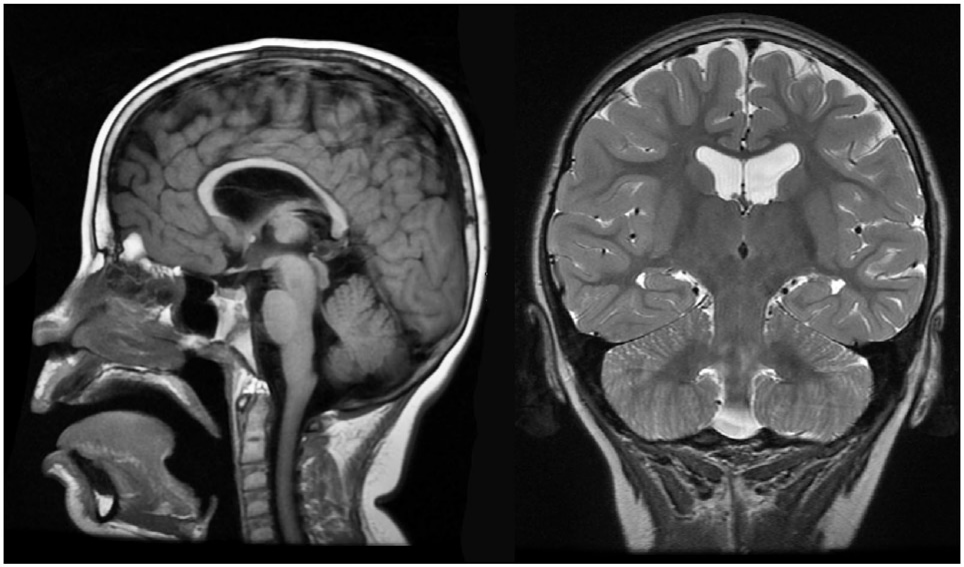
Case 1 (WDR45) high resolution 3T magnetic resonance imaging brain scan. Sagittal T1 fluid attenuated inverse recovery imaging (left) demonstrates thin corpus callosum. T2 coronal imaging (right) demonstrates slight cortical atrophy and slight hippocampal asymmetry.
